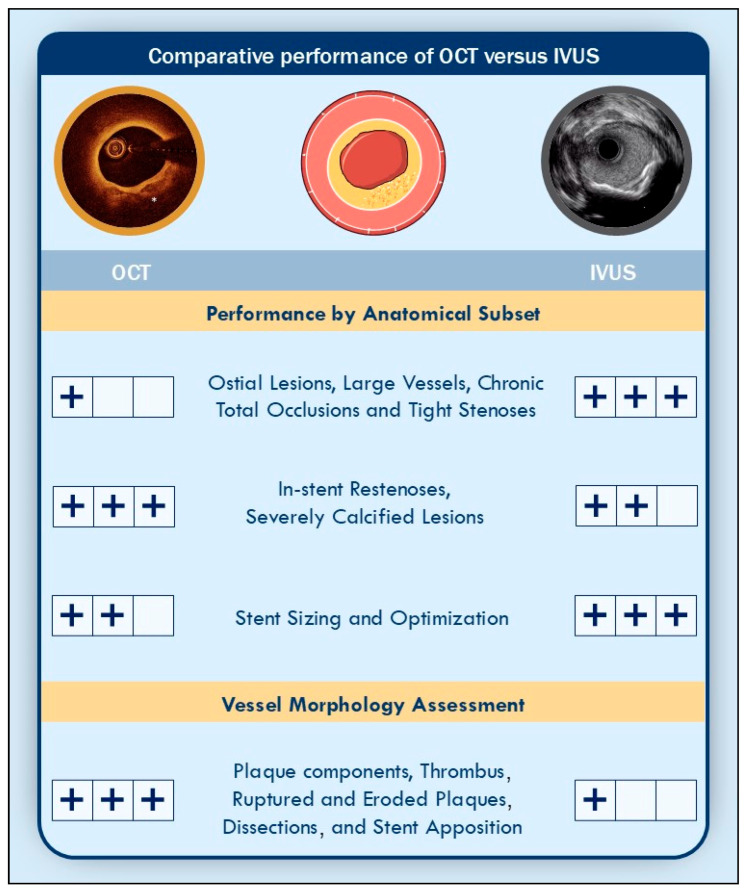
Performance of optical coherence tomography versus intravascular ultrasound by anatomical subset. Abbreviations: IVUS, Intravascular Ultrasound; OCT, Optical Coherence Tomography.
